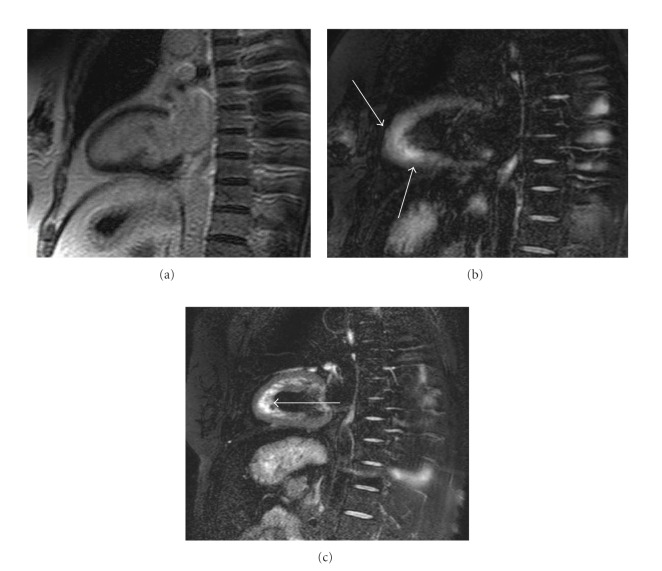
55-year-old female with takotsubo cardiomyopathy. Long-axis delayed enhancement (a) shows no myocardial scarring of the apical myocardium, whereas the T2-weighted MRI (b) shows circumferential myocardial edema at the apical and midventricular regions (arrows). The T2-weighted MRI at its remission (c) shows no myocardial edema. The flow artifact is seen in the apical cavity (arrow), due to the insufficient blood signal suppression by black-blood prepulse.
